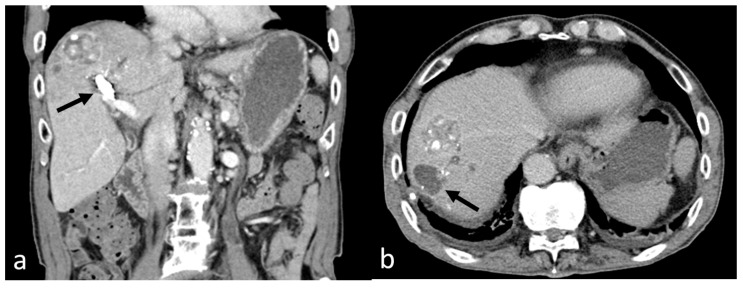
(a) The pseudoaneurysm was successfully embolized (arrow), and hemobilia was resolved as found on the follow-up computed tomography image. (b) However, several bilomas (arrow) were newly observed in segments 5 and 8 of the liver.
